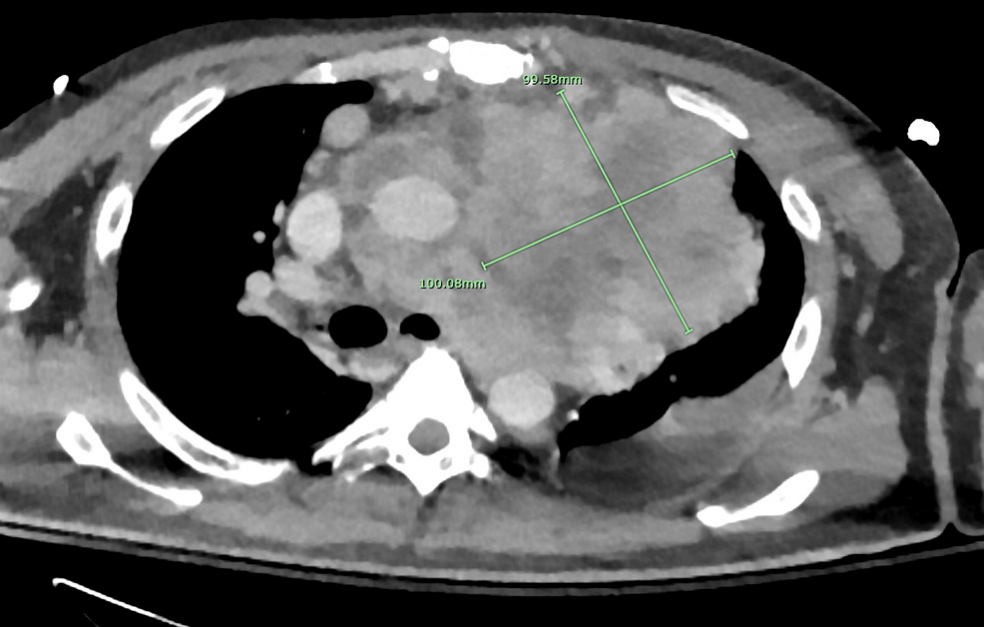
CT scan of the chest with IV contrast showing the mass at diagnosis.
